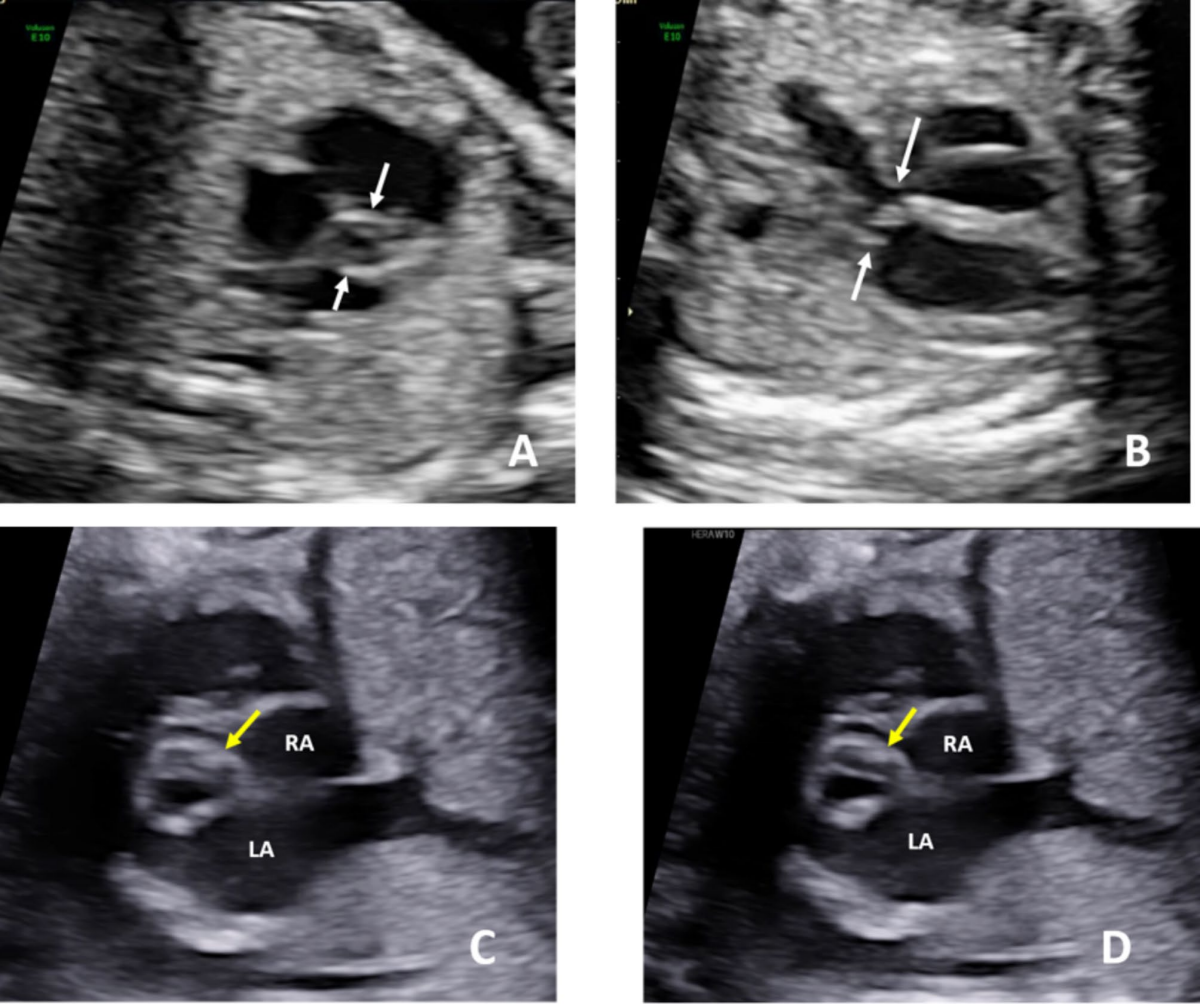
Bicuspid aortic valve in fetal 2-dimensional echocardiogram: The short axis view of the right ventricle shows two commissures and a “fish-mouth” opening (A). The left ventricular outflow tract view shows thickening and hyperechogenicity (B). The short axis view of the right ventricle shows a raphe (C and D)
